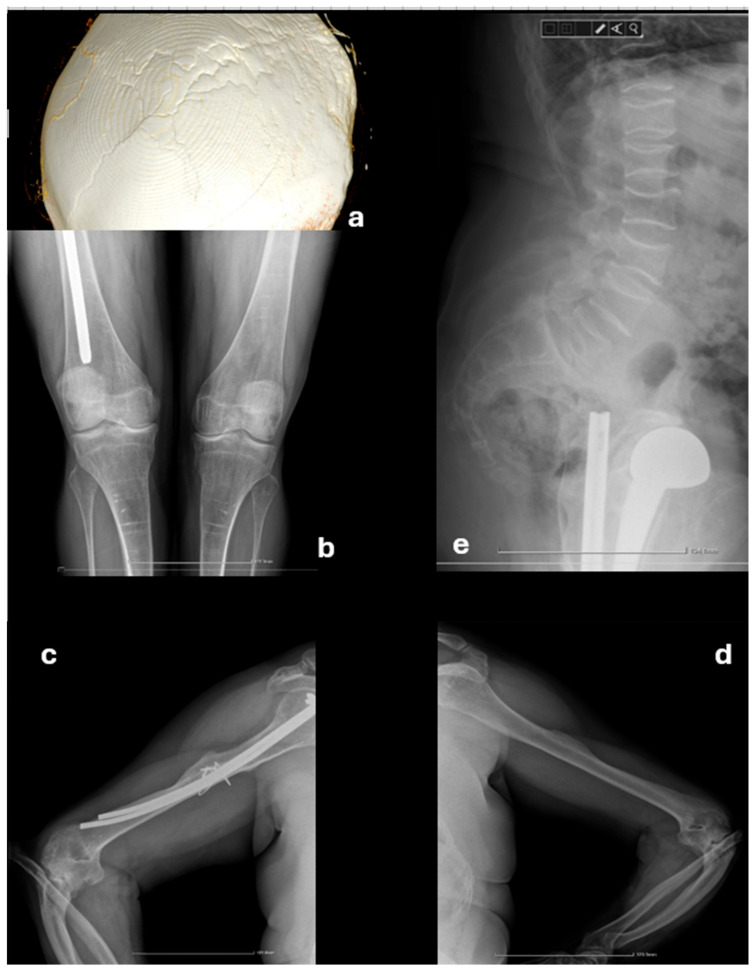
Radiographic investigations. (a) Wormian bones. (b) Bilateral patella alta and osteosclerotic bands in the femoral and tibial diaphysis. (c,d) Bilateral radial head dislocation. (e) Codfish vertebrae in the thoraco-lumbar spine.
